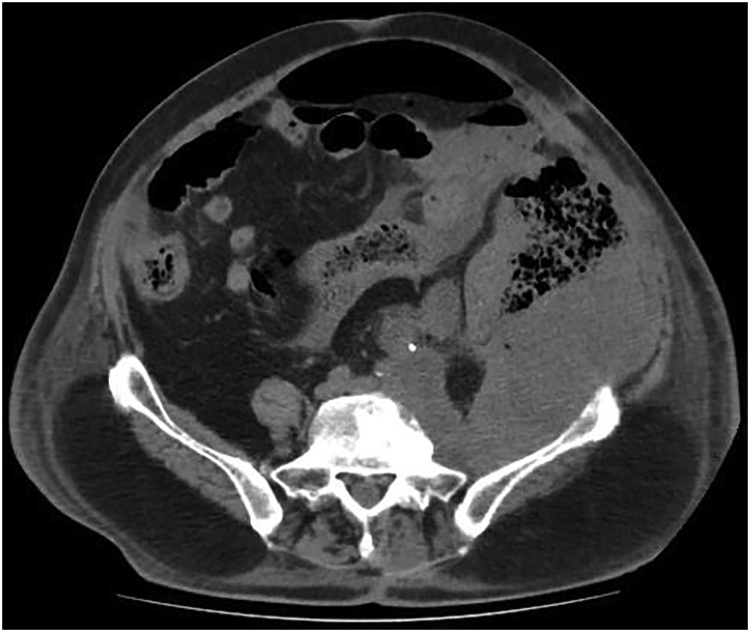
CT revealed the presence of a 16*10*12 cm solid left retroperitoneal mass with infiltration and perforation of the descending colon.
